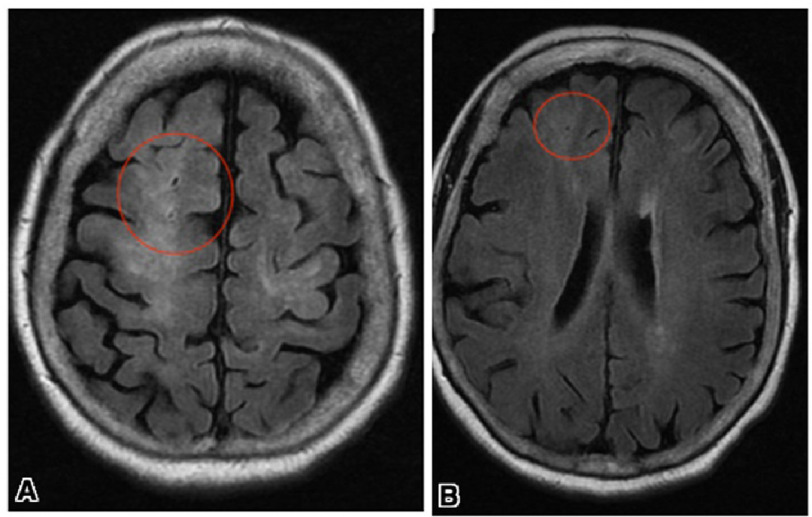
Initial brain MRI showing multifocal areas of ischemia consistent with a central embolic source in a multivessel peripheral distribution, with no evidence of hemorrhage (A). A few foci of air in the sulci within the vessels are evident (A), (B). The etiology of the multiple air emboli was unclear but the air appears to be within the bridging veins in the subarachnoid space.
